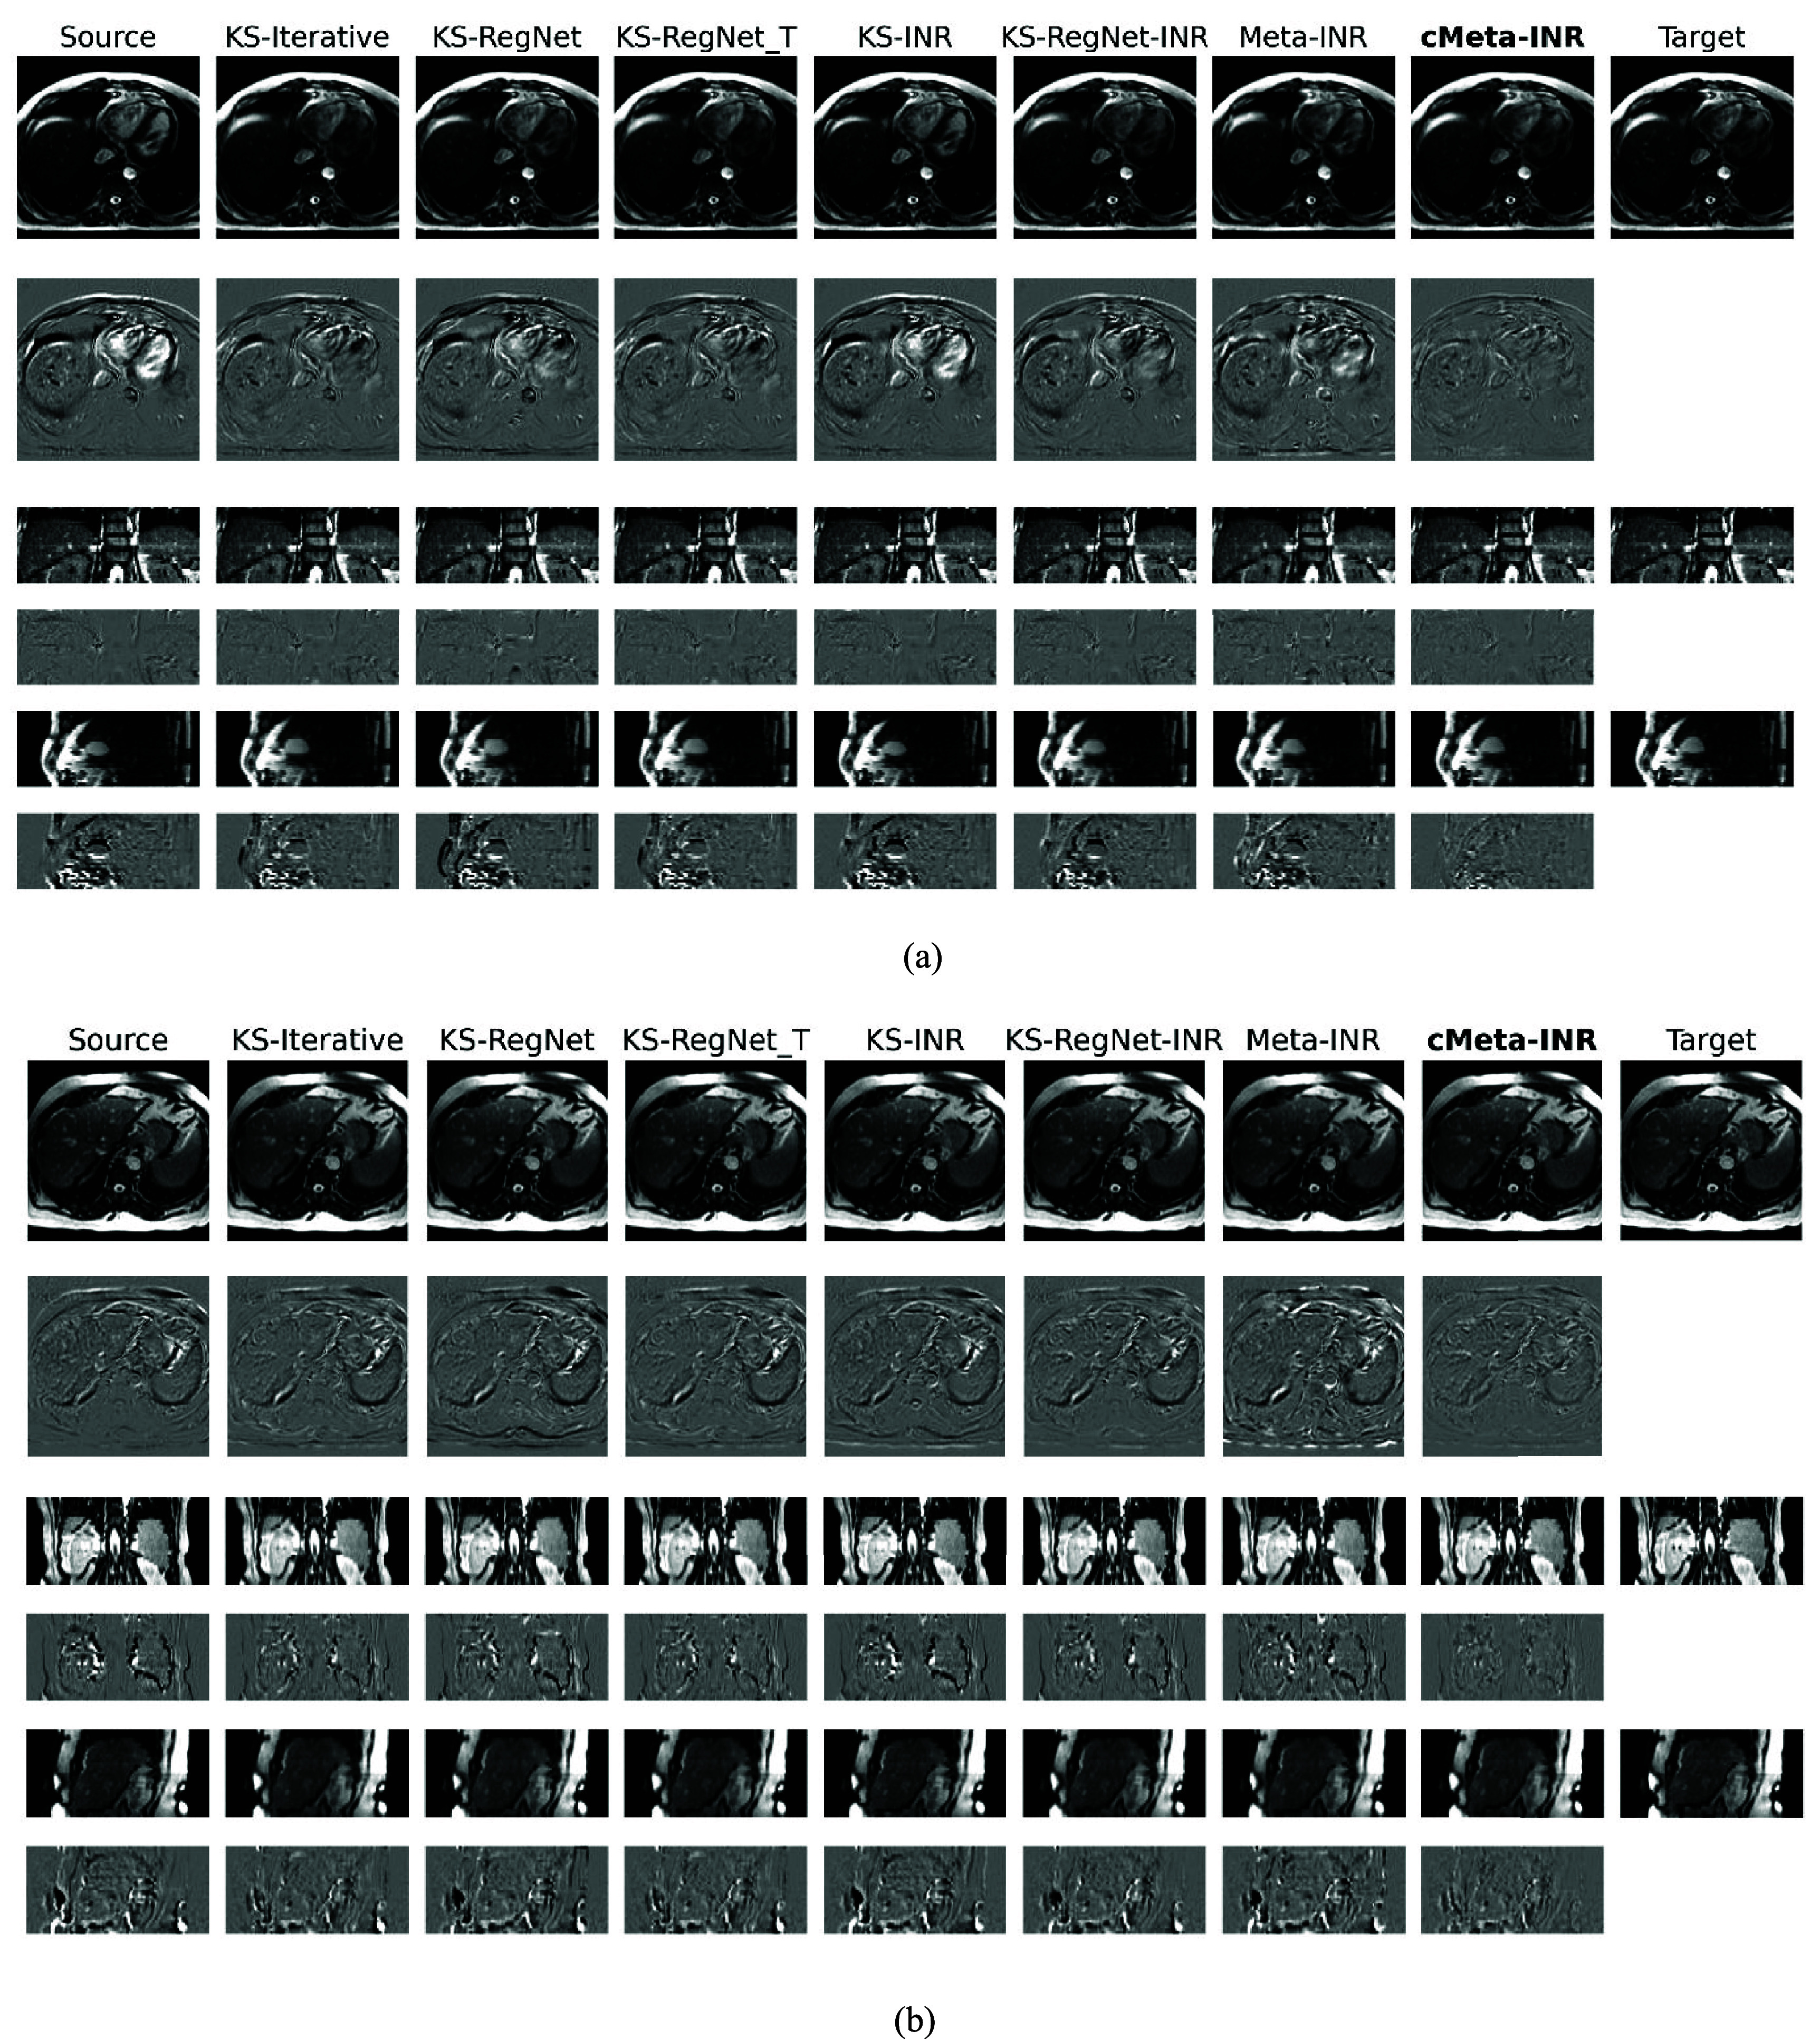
Comparison of abdominal MR images solved by different methods. Subfigures (a) and (b) present the images from different subjects in the axial, coronal, and sagittal views (odd rows), and the differences between these images and the target images (even rows). The spoke number used for *k*-space sampling is 13.
